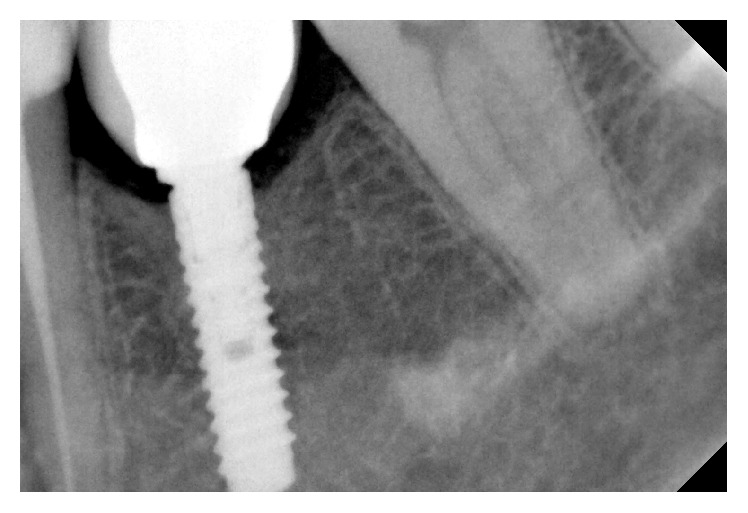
Periapical X-ray immediately after loading.
